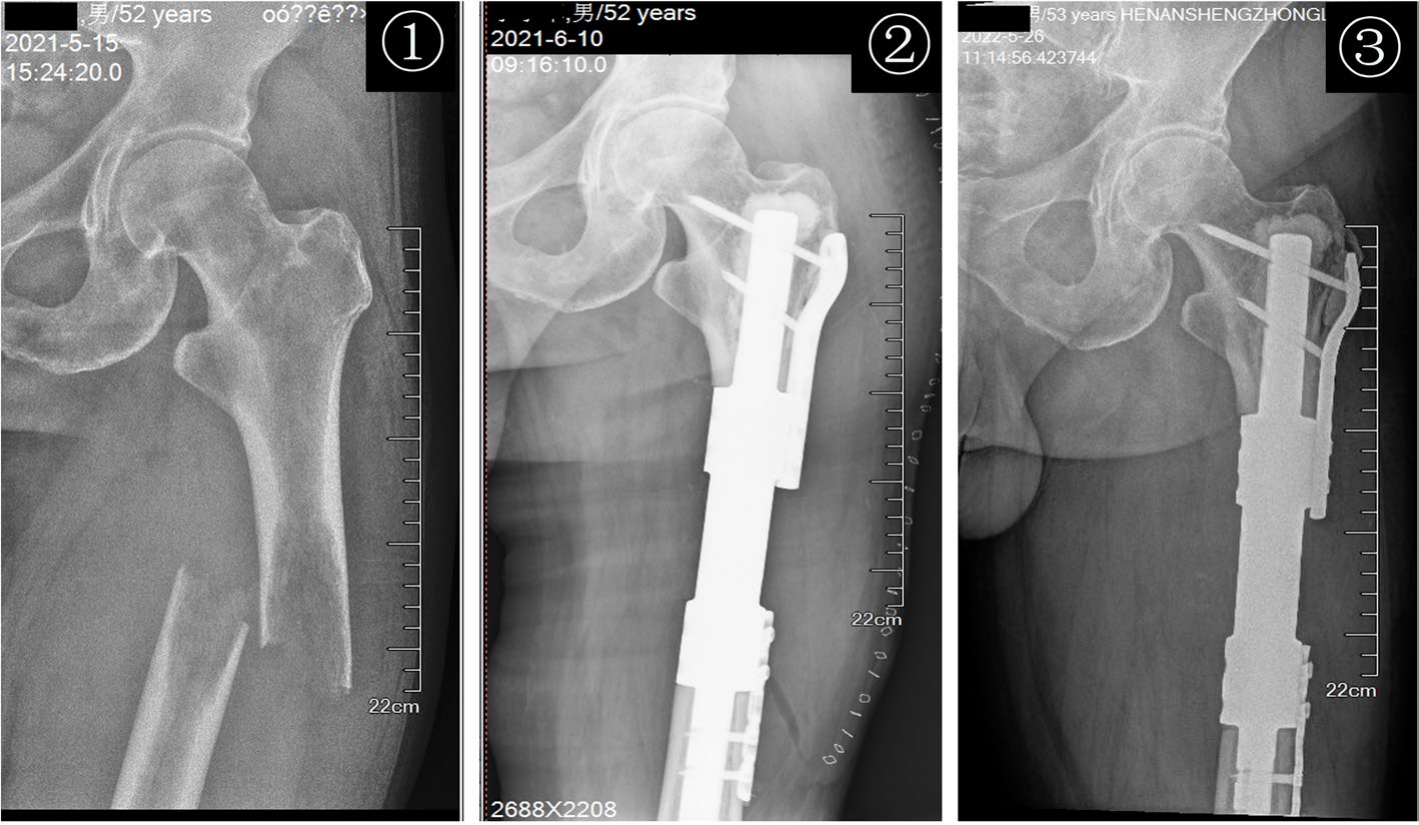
Imaging follow-up process of segmental intercalary prosthesis. ① Positive X-ray of the left femur showing pathological fracture of the shaft due to metastatic tumor, there were osteolytic destruction, discontinuity of bone cortex, obvious fracture line, and angular deformity at the proximal end of the left femoral shaft. ②–③ One month and 12 months after the operation, the positive X-ray films of the proximal femur showed that the prosthesis was in a good position
